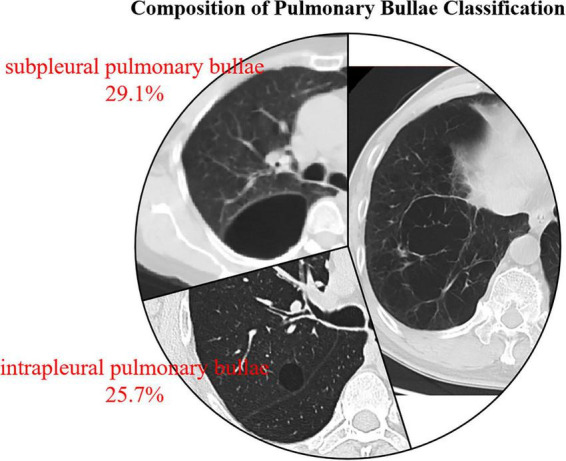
Proportions of different types of pulmonary bullae (created by PowerPoint, Chest CT images of pulmonary bullae were obtained from patients in this study with informed consent.)
